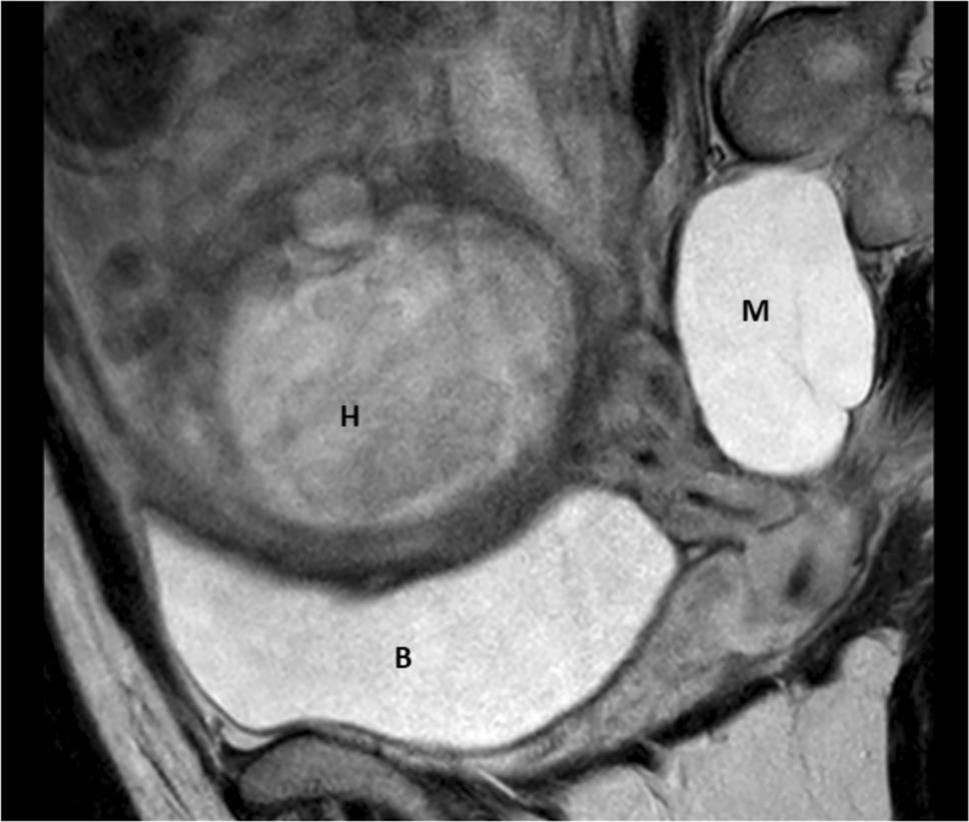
Sagittal T2-weighted MRI image at 30 weeks’ gestation showing right-sided meningocele with only slight indentation by fetal head and uterus M = meningocele, H = fetal head, B = bladder
